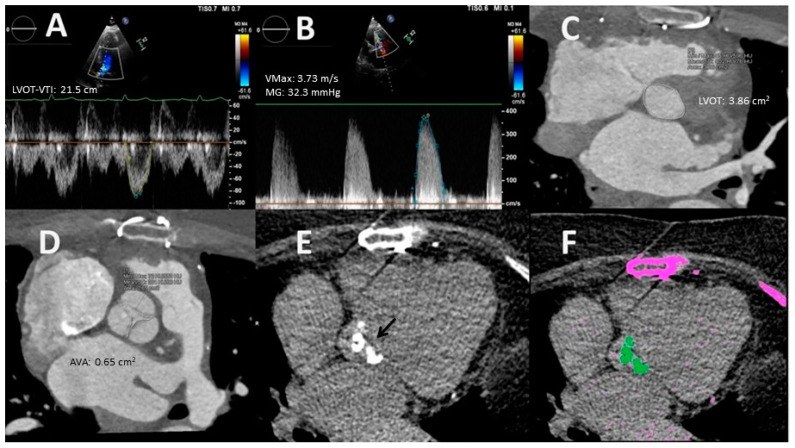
Example of a challenging AS scenario. This 80-year-old woman presented with symptomatic AS, which was initially classified as NF–LG severe AS based on TTE results. (A) A5C view: LVOT–VTI assessment. (B) The best transvalvular flow alignment was obtained from RPV, but VMax was <4 m/s (3.73 m/s). (C) LVOT planimetry by MDTC (3.86 cm^2^). AVA estimated by the hybrid method was 0.92 cm^2^. (D) AV direct planimetry by MDTC (AVA = 0.65 cm^2^). (E) Non-contrast CT image showing calcification of the trileaflet aortic valve (black arrow). (F) AV calcium score quantification; AV calcium is highlighted in green (1332 AU). AS was finally graded as severe. TAVR was performed without complications. The patient remained asymptomatic at two-years follow up. AV = aortic valve; AVA = aortic valve are; AS = aortic stenosis; AU = Agatston units; A5C = apical-5-chambers; CT = computes tomography; LVOT = left ventricular outflow tract; LVOT-VTI = left ventricular outflow tract velocity time integral; MDCT = multidetector cardiac tomography; NF–LG = normal-flow low-gradient; RPV = right parasternal view; TAVR = transcatheter aortic valve replacement; TTE = transthoracic echocardiography; VMax = transvalvular flow maximal velocity.
